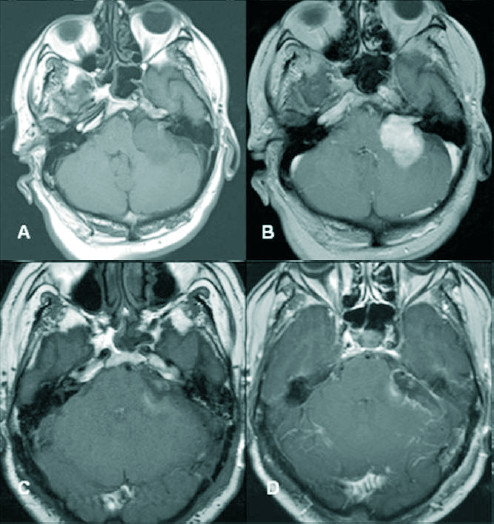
Preoperative (A+B) and postoperative (C+D) MRIs: (A+C)Axial T1Wse without and (B+D)with contrast. MRI findings: Enlargement of the left IAC. In non-contrast T1w homogeneous intermediate signal mass in the CPA-IAC cistern on the left with displacement of the middle cerebellar peduncle and strong enhancement after contrast administration. No intramural cysts and no dural tail. C+D, no residual tumor is shown.
